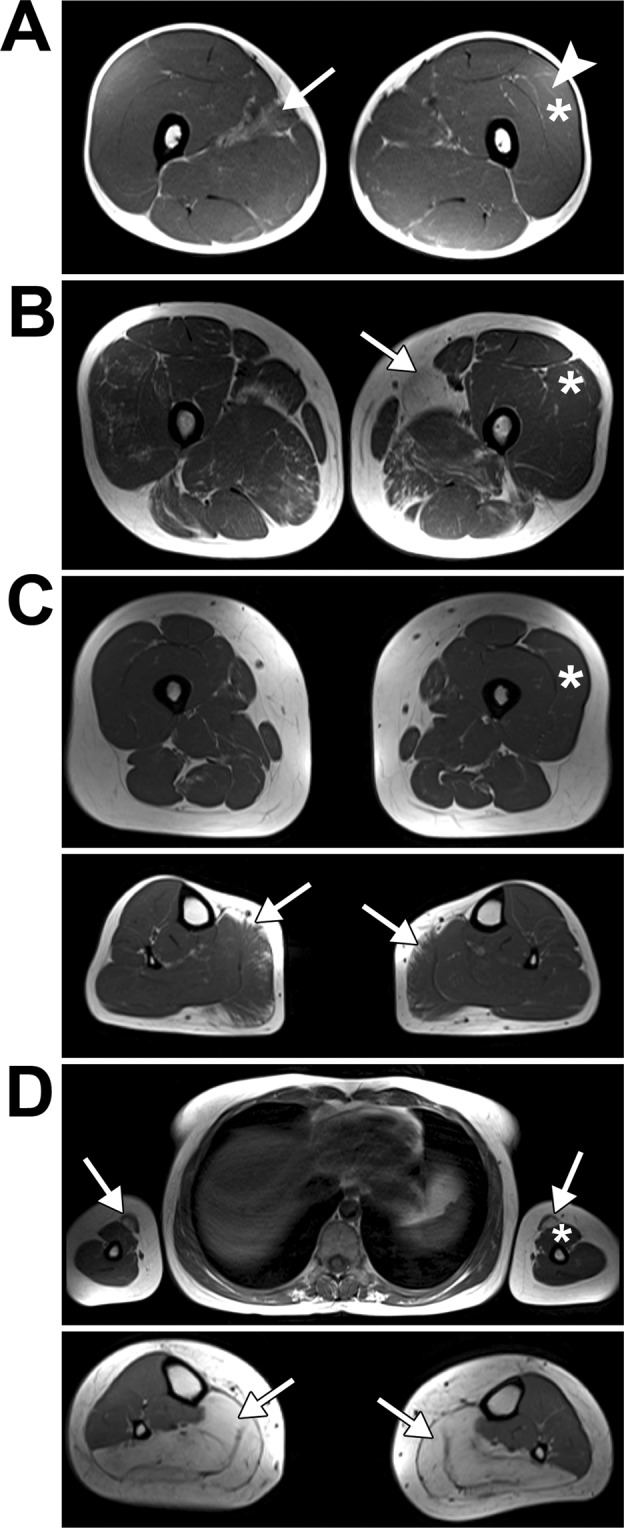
Magnetic Resonance Imaging (MRI). (A–D) Selected axial T1W images of muscles of patients 2–5. (A) Images of patient 2 thigh muscles - arrow points to fatty atrophy in right adductor longus muscle; asterisk marks biopsied left vastus lateralis muscle with a mild fatty degeneration (arrowhead). (B) Images of patient 3 thigh muscles - arrow points to severe fatty atrophy in left adductor longus muscle; asterisk marks biopsied left vastus lateralis muscle. (C) Images of patient 4 muscles; upper panel - thigh muscles with biopsied left vastus lateralis muscle (asterisk); lower panel - arrows point to fatty atrophy in medial head gastrocnemius muscles. (D) Images of patient 5 muscles; upper panel - arrows point to fatty infiltrations in biceps brachii muscles, asterisk marks biopsied left biceps brachii muscle; lower panel - arrows point to severe bilateral fatty infiltration of the soleus and gastrocnemius muscles.
